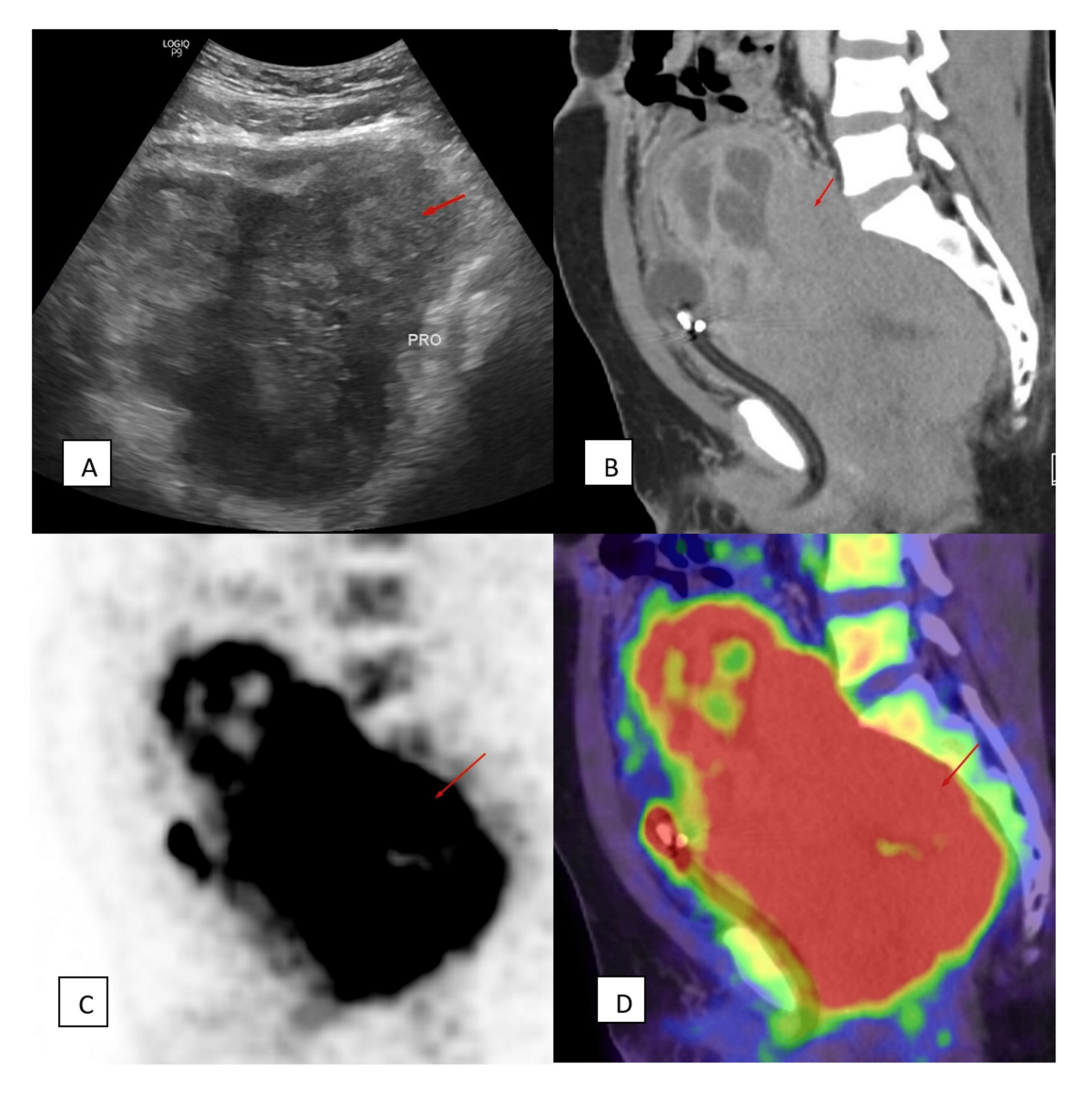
(A) Ultrasound, (B) PET-CT images of the prostate mass demonstrating heterogeneous echotexture, (C) diffuse post-contrast enhancement, and (D) avid FDG uptake. Ultrasound shows a grossly enlarged prostate with heterogeneous echotexture (A). Grossly enlarged prostate with heterogeneous post-contrast enhancement (C). On PET-CT images, the enlarged prostate shows diffuse avid FDG uptake (D). FGD: fluorodeoxyglucose
